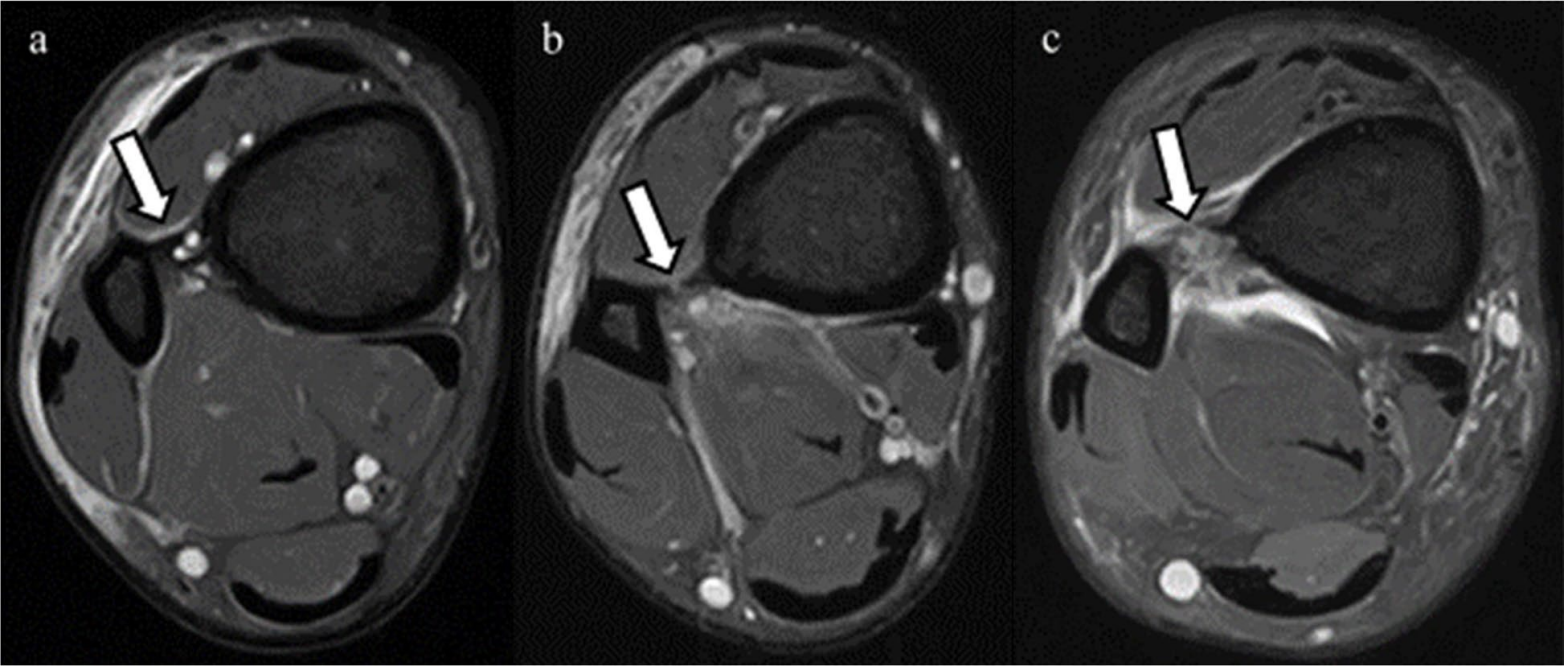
Interosseous ligament (IL) in oblique axial proton-density fat saturation MRI. The arrows in the figures indicate IL. a The intact IL. b Partial tear of the IL, with complete discontinuity in this slice, but continuity is visible in the other slices. c Complete tear of the IL, showing complete discontinuity
